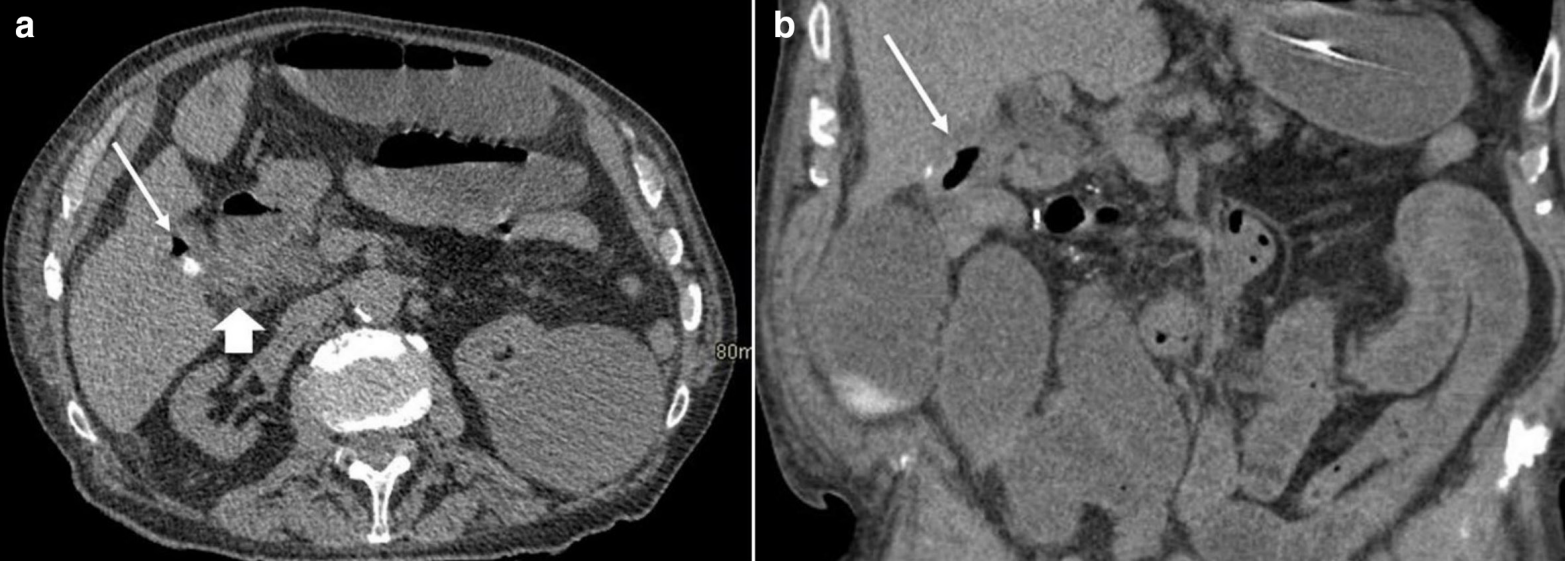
(a, b) Coronal and axial sections at the level of the upper abdomen on the presentation CT, demonstrating gas and a small calcified gallstone within a thick-walled gallbladder (arrowed). Also noted are dilated small bowel loops. There is loss of fat plane between the gallbladder and the second part of the duodenum with surrounding fat stranding suggestive of the site of cholecystoduodenal fistula (arrow head).
